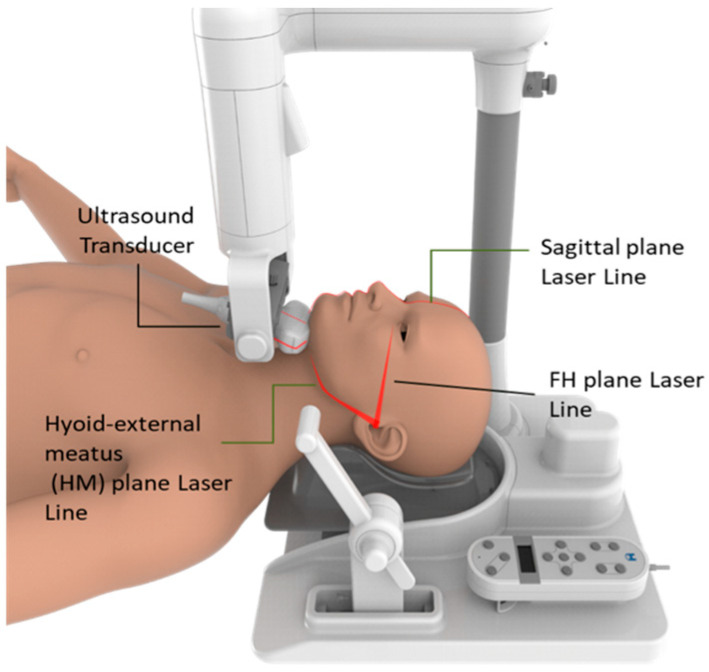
Submental ultrasonography equipment, laser alignment (AmCad BioMed Corporation).
